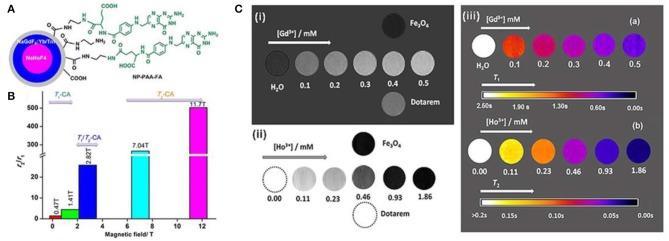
(A) Atomic structure of prepared NP-PAA-FA; (B) the varied r_2_/r_1_ as a function of magnetic field; (C) (i) shows the T_1_-weighted MRI; (ii) shows the T_2_-weighted MRI; (iii) shows the maps of solution with NP-PAA-FA at magnetic field of 3.0 T (Biju et al., 2018); Copyright 2018, reproduced with permission from John Wiley and Sons.
